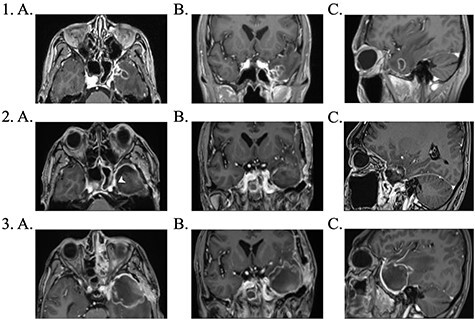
1. Pre-operative contrast enhanced brain MRI: (a) T1 axial view with enhancing lesion; (b) T1 coronal and (c) T1 sagittal. 2. First post-operative contrast enhanced brain MRI showing resection of lesion: (a) T1 axial. Arrow: dural enhancement of sphenoid sinus; (b) T1 coronal and (c) T1 sagittal. 3. Second post-operative contrast enhanced brain MRI following endoscopic re-resection: (a) T1 axial; (b) T1 coronal and (c) T1 sagittal.
